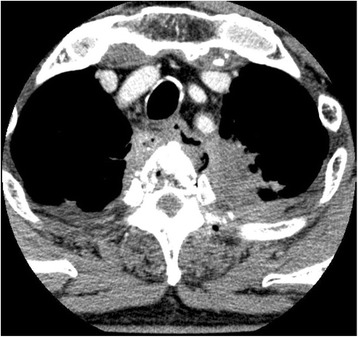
Contrast-enhanced chest CT scan suggested the perforation of the esophagus in the upper left lateral wall with fluid and air in the posterior mediastinum. Osteolytic changes with free air space were observed within the vertebral body
